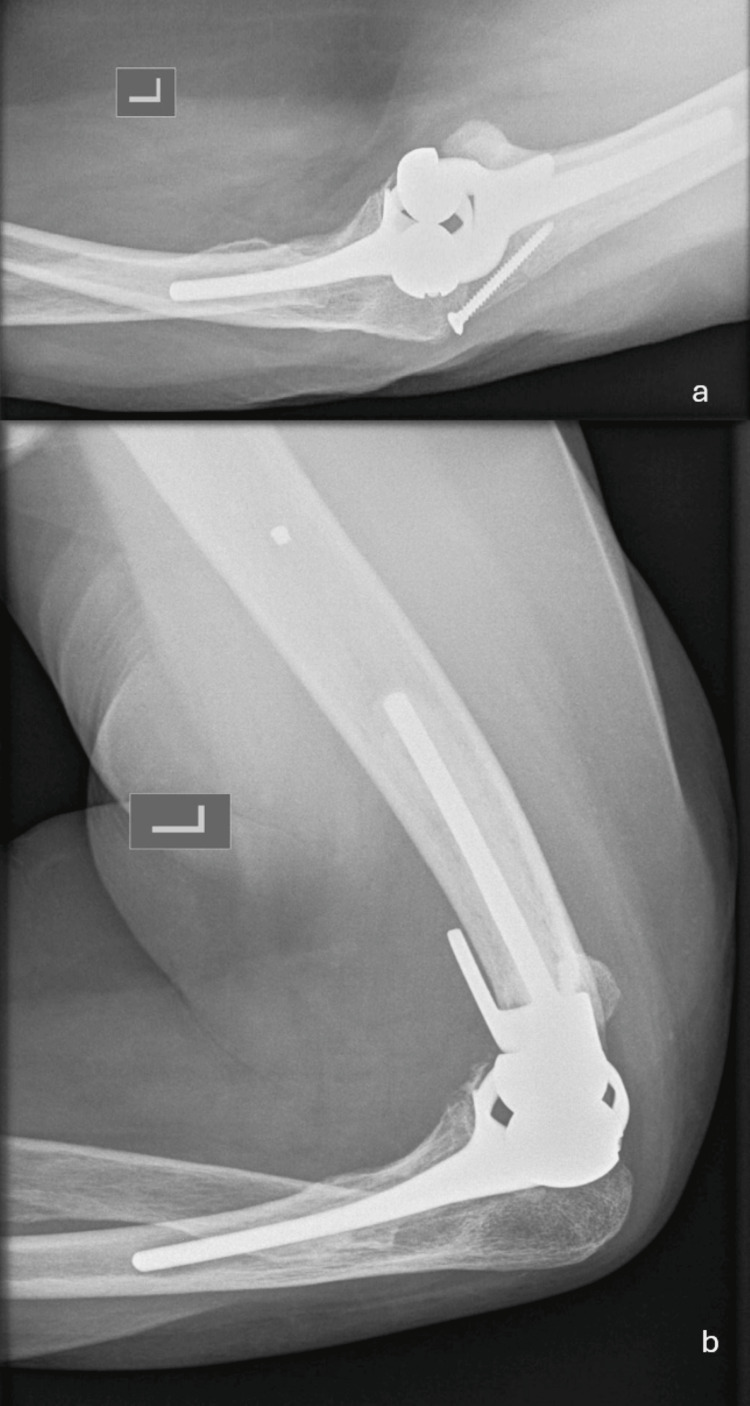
(a) Anteroposterior and (b) lateral X-rays at the six-month follow-up.
